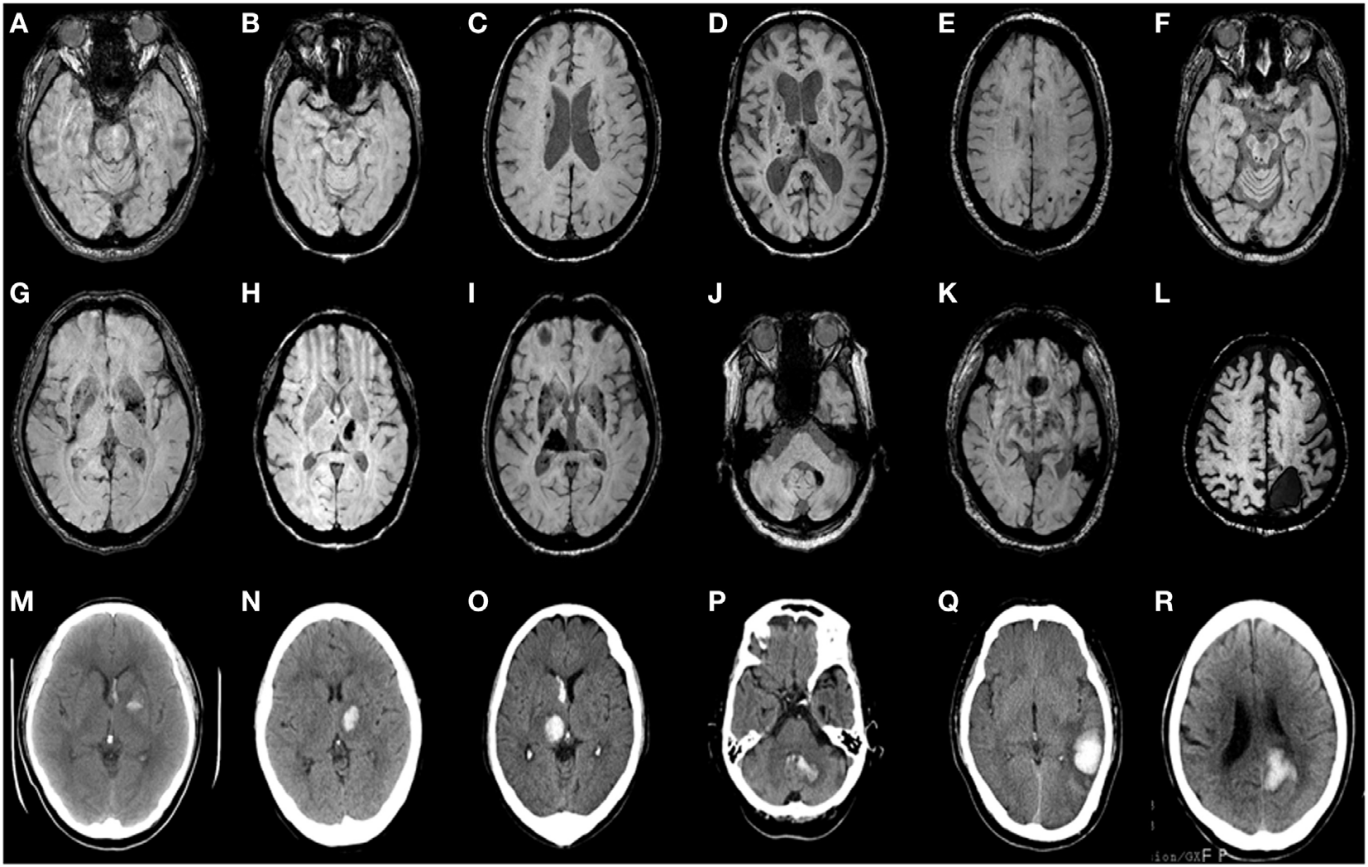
Axial brain CT and matching susceptibility-weighted images (SWI) of six CADASIL patients with intracerebral hemorrhage (ICH) (A–R). SWI (A–F) shows cerebral microbleeds without ICH. SWI (G–L) and matching unenhanced brain CT (M–R) demonstrate acute ICH with or without intraventricular hemorrhage. Panels (A,G,M) are patient 1. (B,H,N) are patient 2. (C,I,O) are patient 3. (D,J,P) are patient 4. (E,K,Q) are patient 5. (F,L,R) are patient 6.
